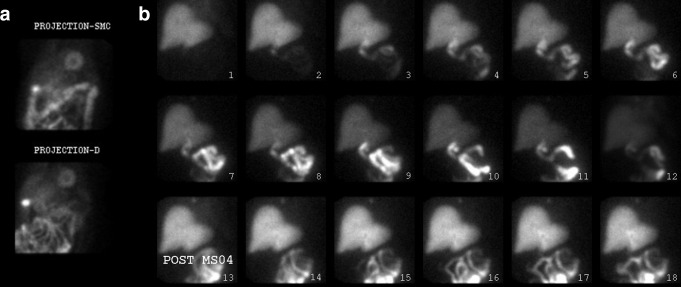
GB visualized on the stress/rest MIBI (a), not on the HBS data even post MS04 and a booster dose (b). GB, gall bladder; HBS, hepatobiliary scintigraphy; MIBI, methoxy isobutyl isonitrile.
